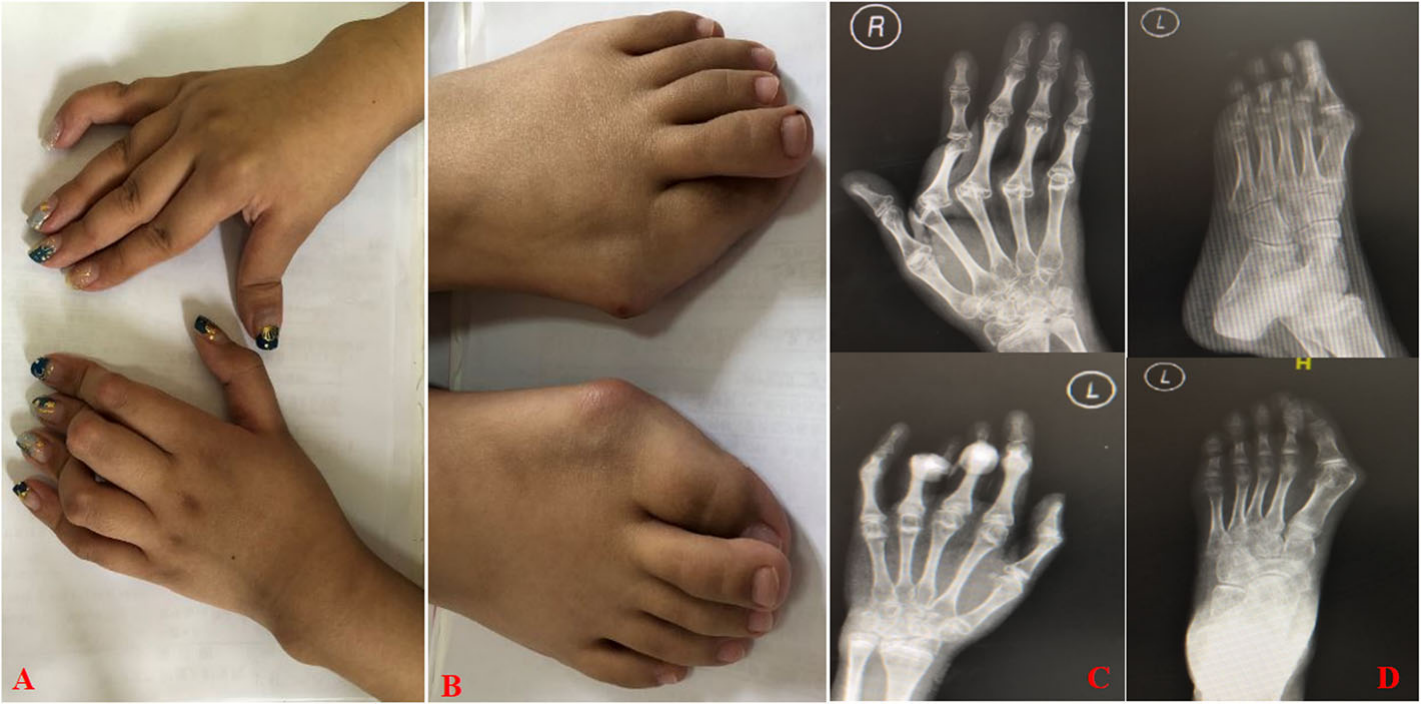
a shows deformities in the proximal interphalangeal joints and wrists, and b shows deformities in the metacarpophalangeal joints and ankles; c reveals deformities of left and right hands, and d reveals deformities of left foot form positive and lateral position by X-rays
